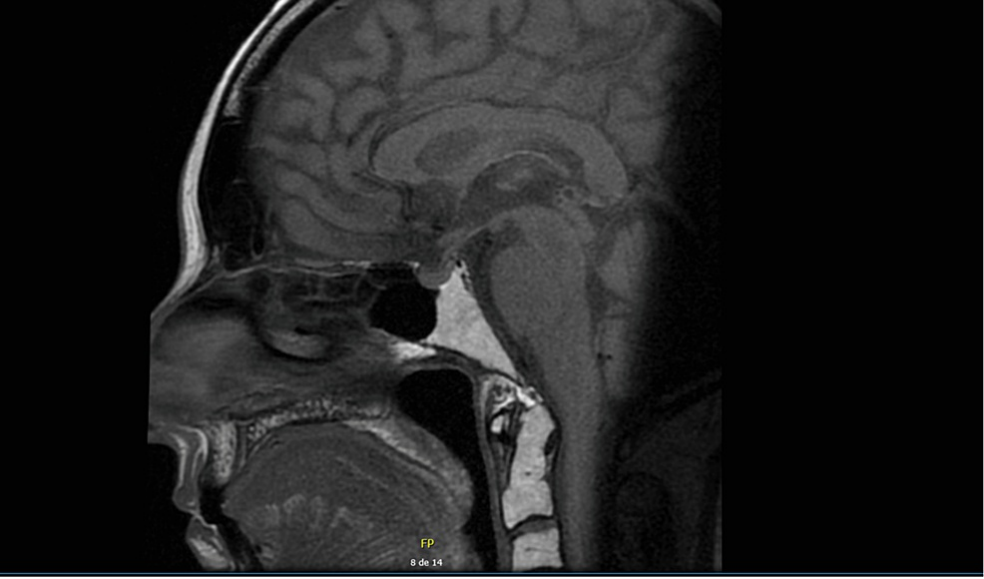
Sagittal view of pituitary MRI (T1-weighted) MRI, magnetic resonance imaging
